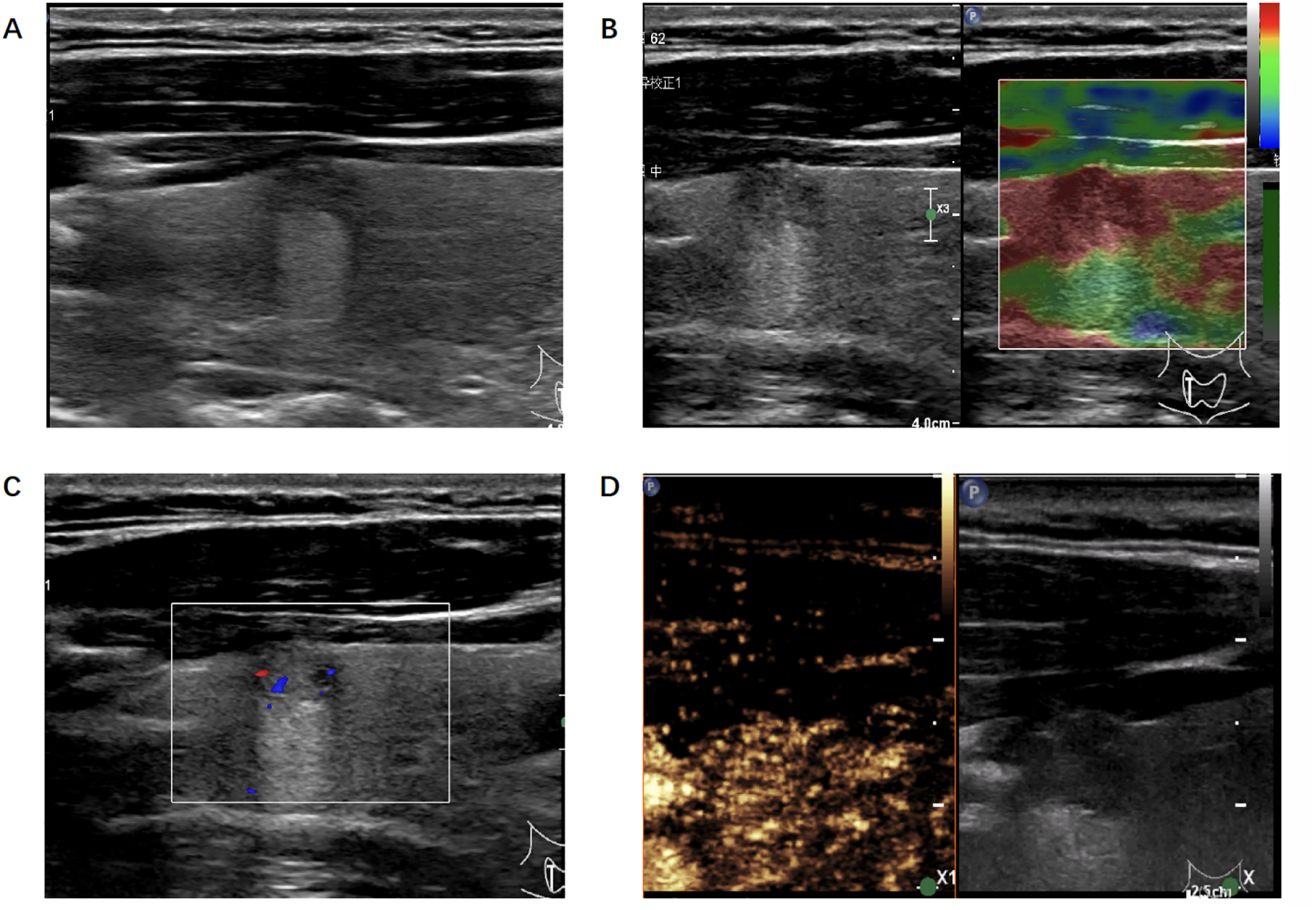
Ultrasonic image of the thyroid nodule which was misjudged by the radiologists but correctly predicted by the algorithm. (A) B-mode ultrasound showed a longitudinal section of the nodule. (B) Real-time elastography image of the nodule. (C) Color doppler flow image of the nodule. (D) The 30-second image of contrast-enhanced ultrasound in thyroid nodule.
